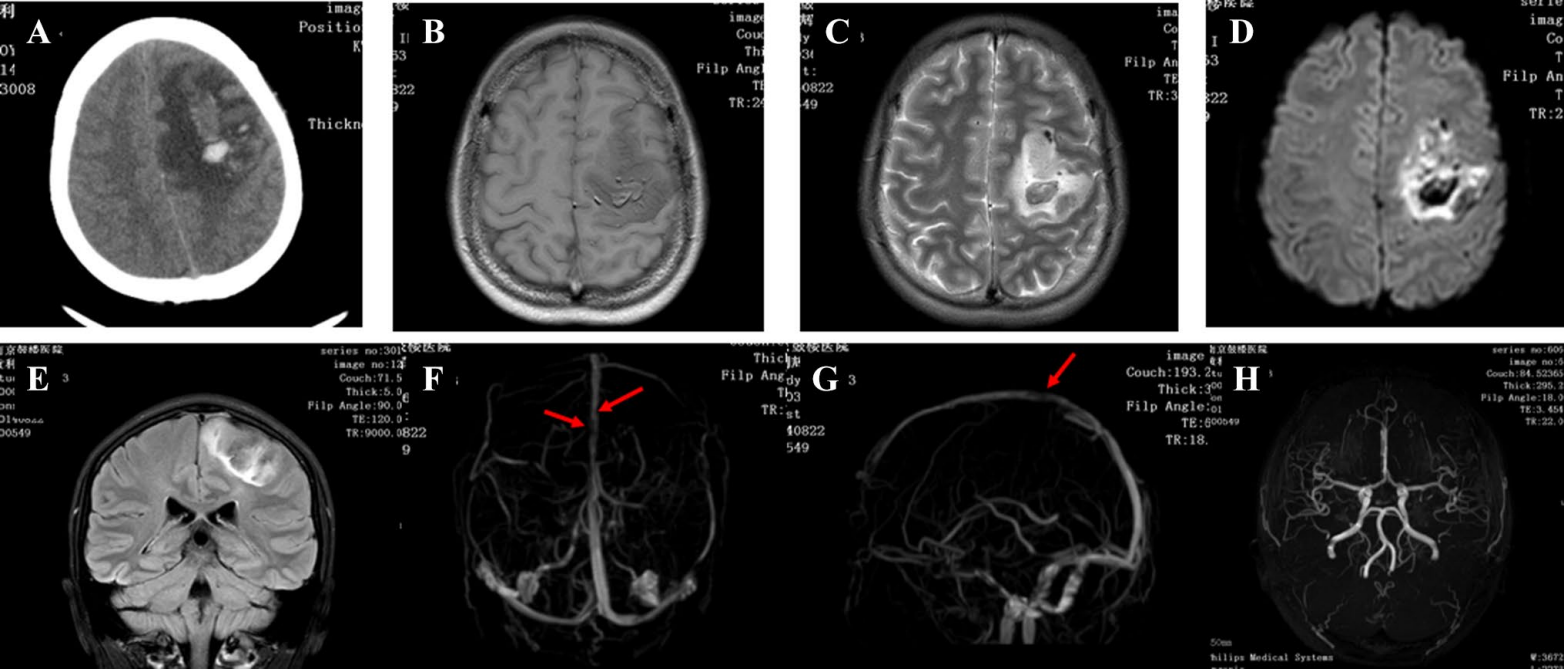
Imaging findings of a representative patient with CVST and concurrent epileptic seizures. a Brain CT scan showing left frontal lobe hemorrhage accompanied by surrounding white matter edema. b T1-weighted MRI showing left-sided intralesional bleeding. c T2-weighted MRI showing intralesional bleeding, edema and mixed signals on the left side. d Diffusion-weighted MRI showing mixed high signals. e T1-weighted coronal MRI showing mixed signals in the left frontal lobe and edema around the lesions. f and g MR venography showing filling defects in the sagittal sinus (*red arrows*). h MR angiography showing no abnormalities in the intracranial arteries
